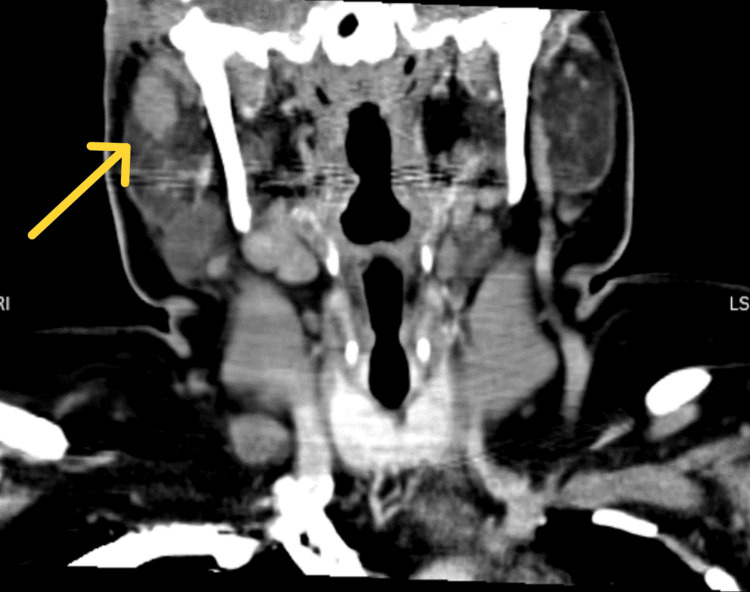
CT of the head and neck (coronal view) showing involvement of the parotid gland.
